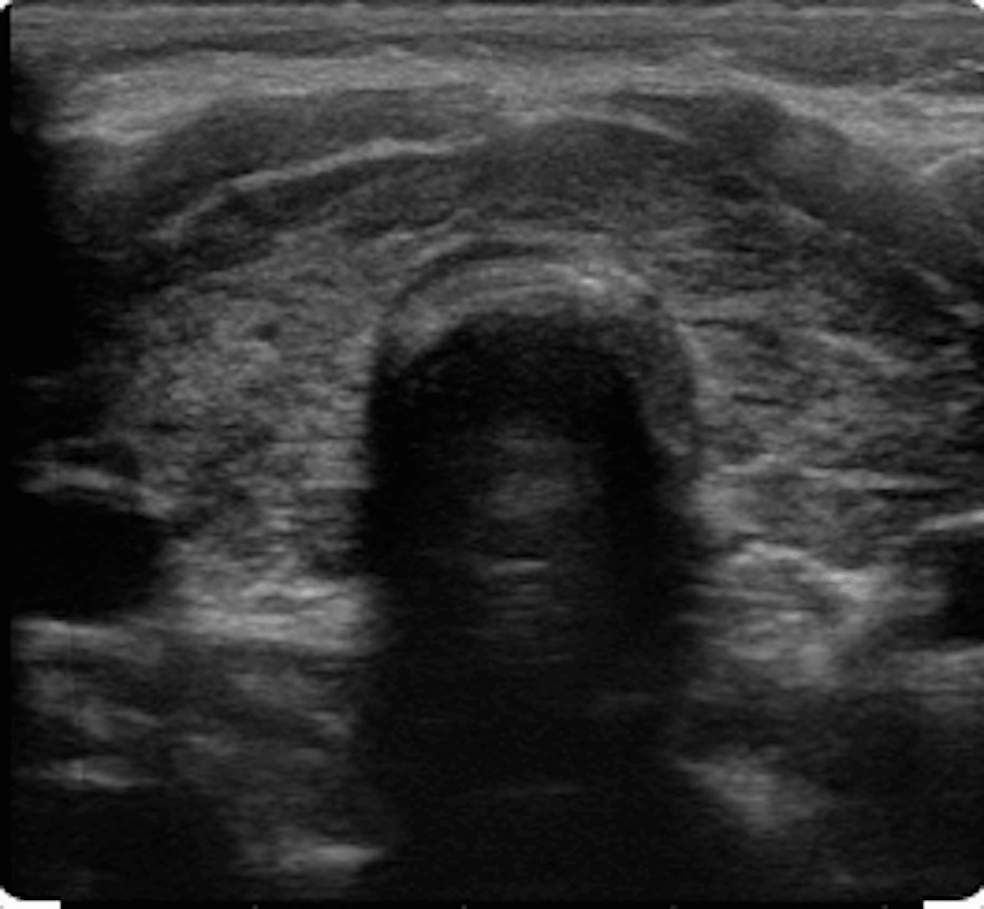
Thyroid ultrasound The image shows a heterogeneous thyroid gland, with a size of 40 mL
